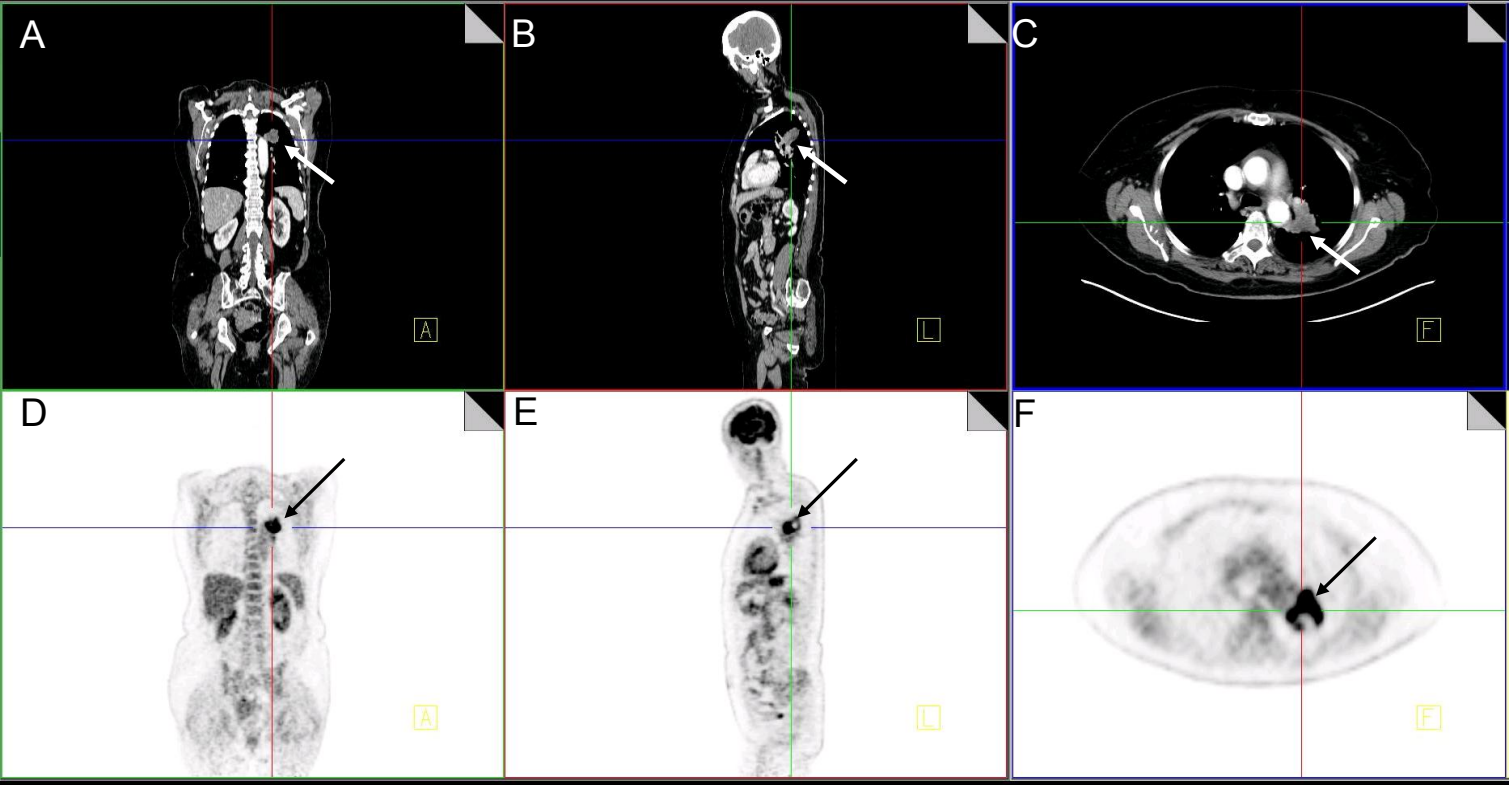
PET image of a patient with a left central lung carcinoma (arrows). Note also the high FDG uptake by the kidneys (Fig D), brain and myocard (Figure E). Source: PET/CT Imaging Centre, University Hospital of Würzburg.
